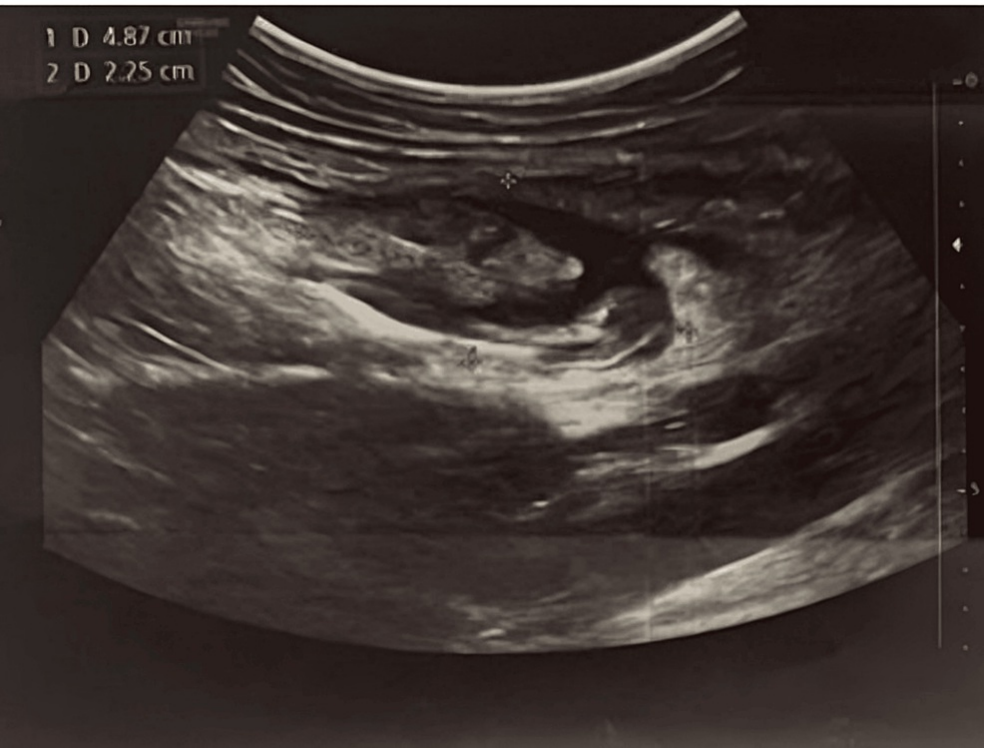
Preoperative abdominal ultrasound shows a collection measuring 48 x 22 mm in the left iliac region.
